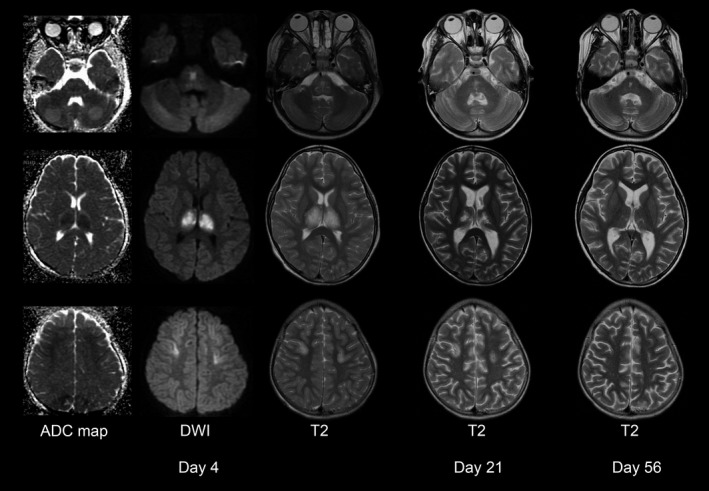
Chronological brain MRI changes. Brain MRIs obtained on day 4 show symmetrical DWI/T2 hyperintensities in the thalamus, frontal cortex, and pons. These brain lesions gradually resolved on day 21 and 56. Note reduction in ADC value in the bilateral thalamic lesions but not apparent changes in other small lesions on ADC map. ADC, apparent diffusion coefficients; DWI, diffusion‐weighted imaging.
